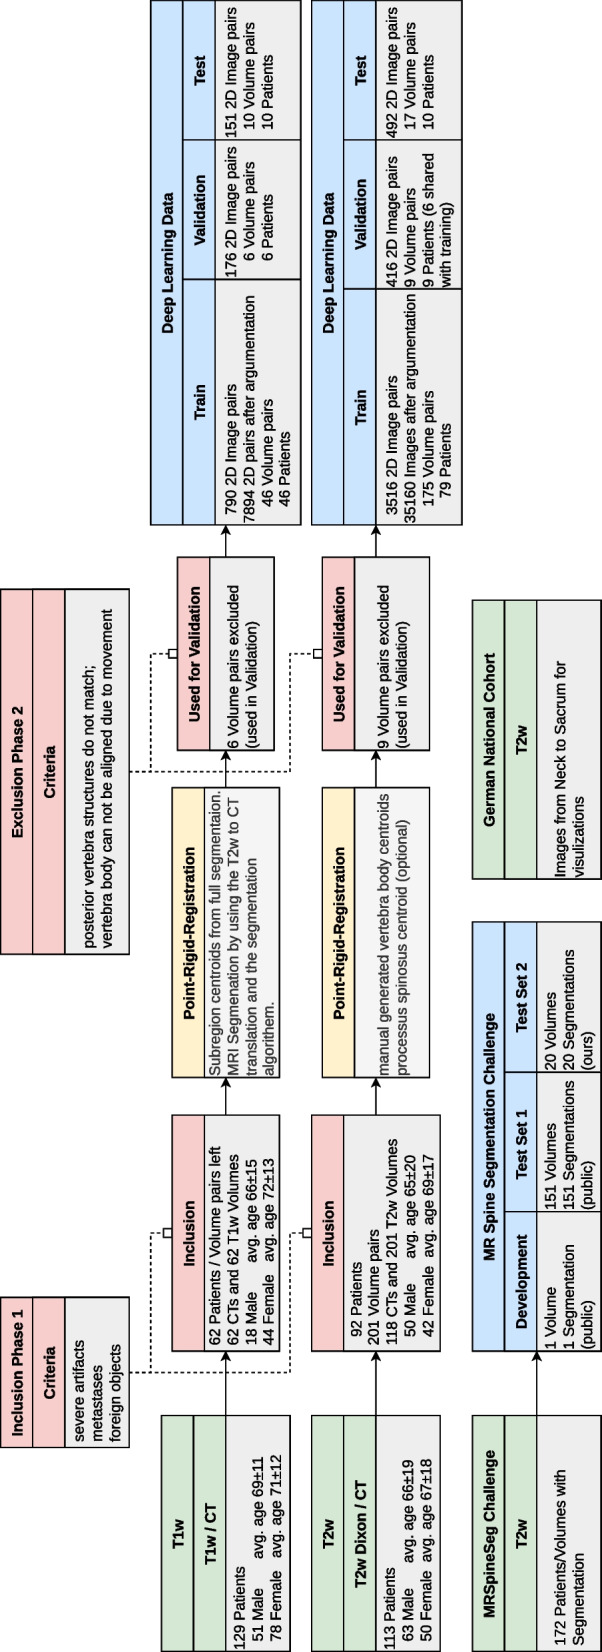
Datasets, preparation, exclusion, and split. MRI data were acquired with 12 different scanners from 3 different vendors. Additionally, we used the MRSSegClg for external testing. For the 2D training, we only consider 2D slices containing a spine. We demonstrated generalizability using a full-body MRI from the German National Cohort dataset for the figures in this paper
